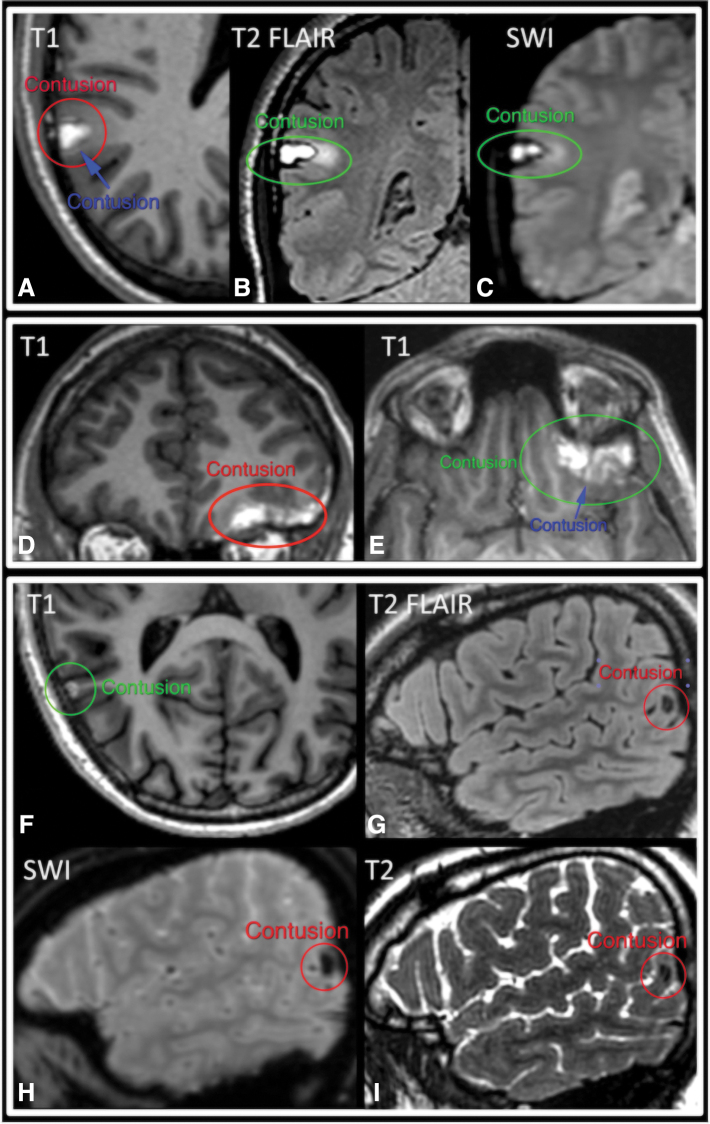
Brain contusion. Two examples of unanimous (A–C, D,E) and one example of partial agreement (F–I) across three neuroradiologists. “Partial agreement” refers to an MRI exam for which the three readers' initial interpretations regarding presence versus absence of a contusion on the exam were not unanimous. Annotations from independent readers are shown in different colors (red, green, and blue) superimposed on the same image for demonstration purposes. Brain contusions generally demonstrate high T1 signal in the 2-week subacute time frame (A, D–F), and they are typically very conspicuous against the lower T1 signal of normal brain and cerebrospinal fluid. *Early* subacute brain contusions contain intracellular methemoglobin and demonstrate very low signal on T2-weighted FLAIR (G), T2*-weighted series (SWI) (H), and T2-weighted series (I), whereas *late* subacute contusions contain extracellular methemoglobin and demonstrate high internal T2 signal (B) and a low-signal-intensity rim of hemosiderin on the T2*-weighted SWI series (C). FLAIR, fluid-attenuated inversion recovery; MRI, magnetic resonance imaging; SWI, susceptibility-weighted imaging.
